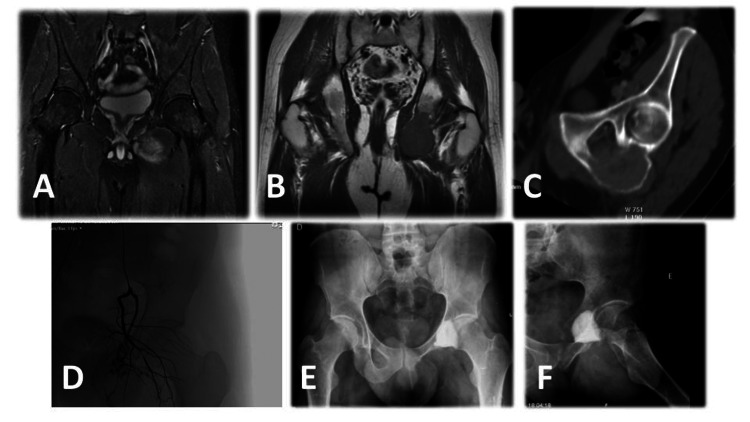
Radiological study of the first patient (A) preoperative MRI coronal STIR sequence; (B) preoperative coronal T1 weighted MRI; (C) preoperative CT scan; (D) preoperative angioembolization; (E) pelvis AP view; (F) frog-leg view postoperative radiographs showing ischiopubic ramus resection and subchondral bone support with cement. STIR: short tau inversion recovery; AP: anteroposterior
